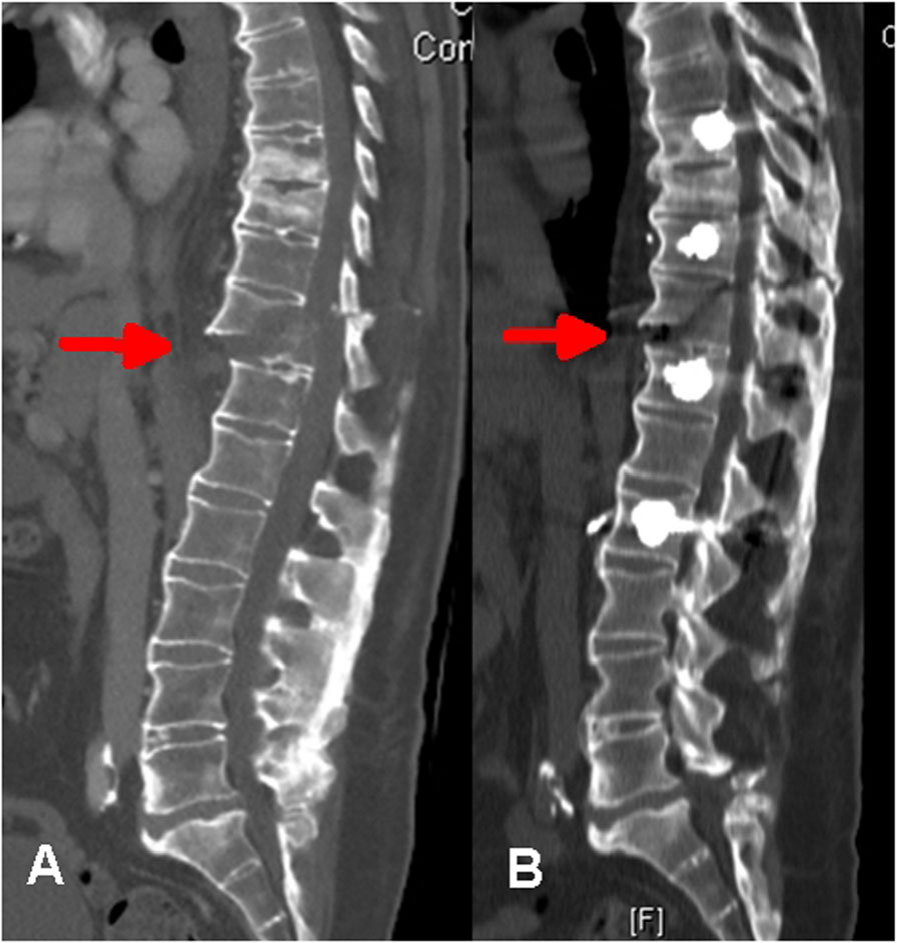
Patient 1, preoperative (labeled a) and postoperative (labeled b) CT sagittal reconstruction. The fracture (marked with red arrows) has reduced, with no residual translation and much less extension
